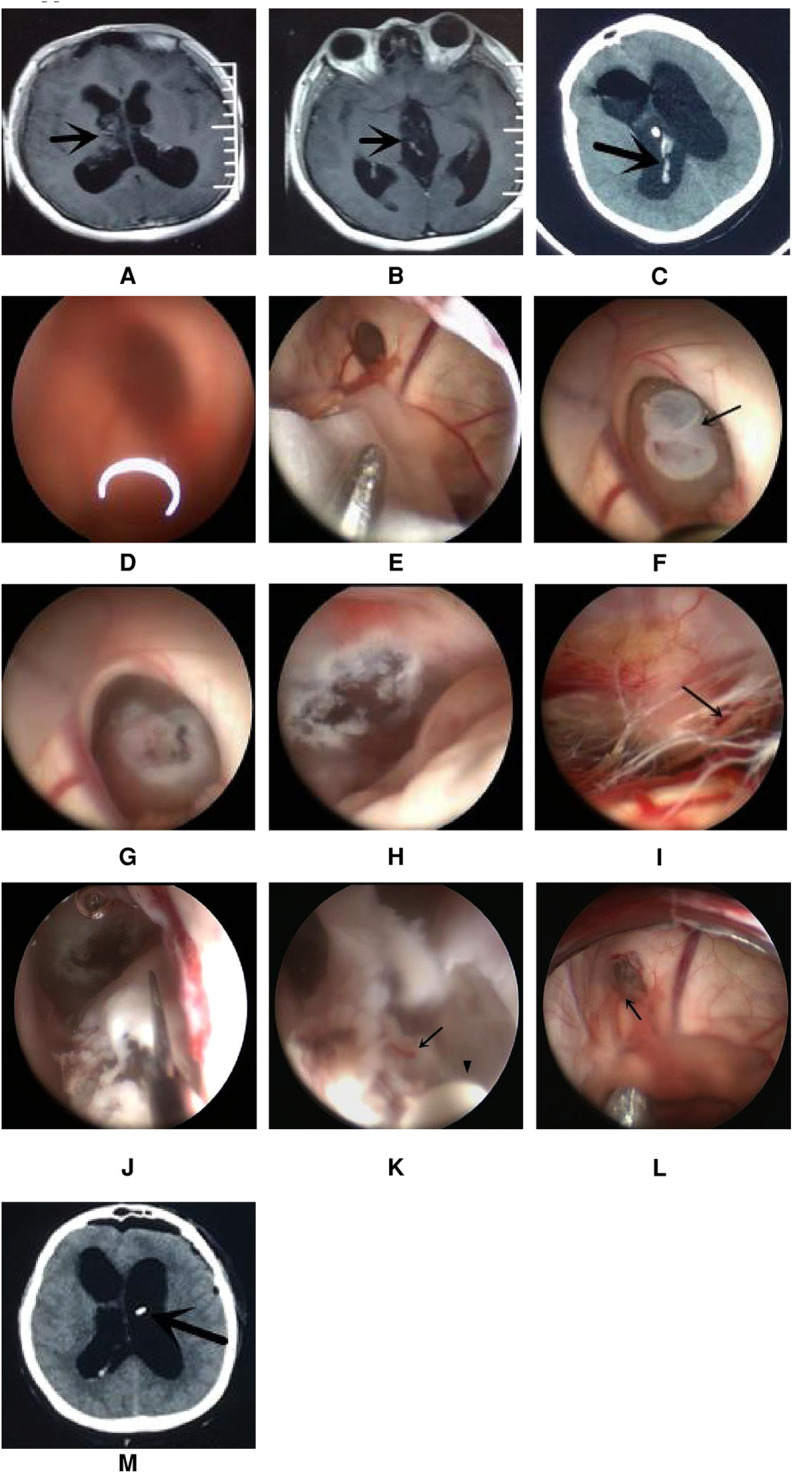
Endoscopic intraventricular surgery was used to perform biopsy and ETV in case 8. (A,B) show MRI scans of the lesion in the right lateral ventricle and third ventricle. (C) CT scan showing a hemorrhage in the ventricle after EVD. (D) Surgical image through the left ventricle showing a blurred image due to a bloody CSF. (E) The extra-endoscopic technique was used to clean the image through a slim pledget and suction. (F) The lesion was seen to block the foramen of Monro. (G) The intra-endoscopic technique was used to coagulate and shrink the tumor. (H) Image showing successful ETV with an orifice of more than 5 mm. (I) Image showing the prepontine cistern; the arrow shows the abducent nerve. (J) The tumor was removed by scissors after ETV. (K) Bleeding (arrow point) from a small artery during continuous irrigation at the course of coagulation of concentric circle bipolar (indicated by the arrowhead). (L) The source of bleeding (arrow point) was located to a vein close to the foramen of Monro and was stopped by the application of the extra-endoscopic technique. (M) CT scan on day 1 after ETV; the arrow shows the Ommaya tube.
